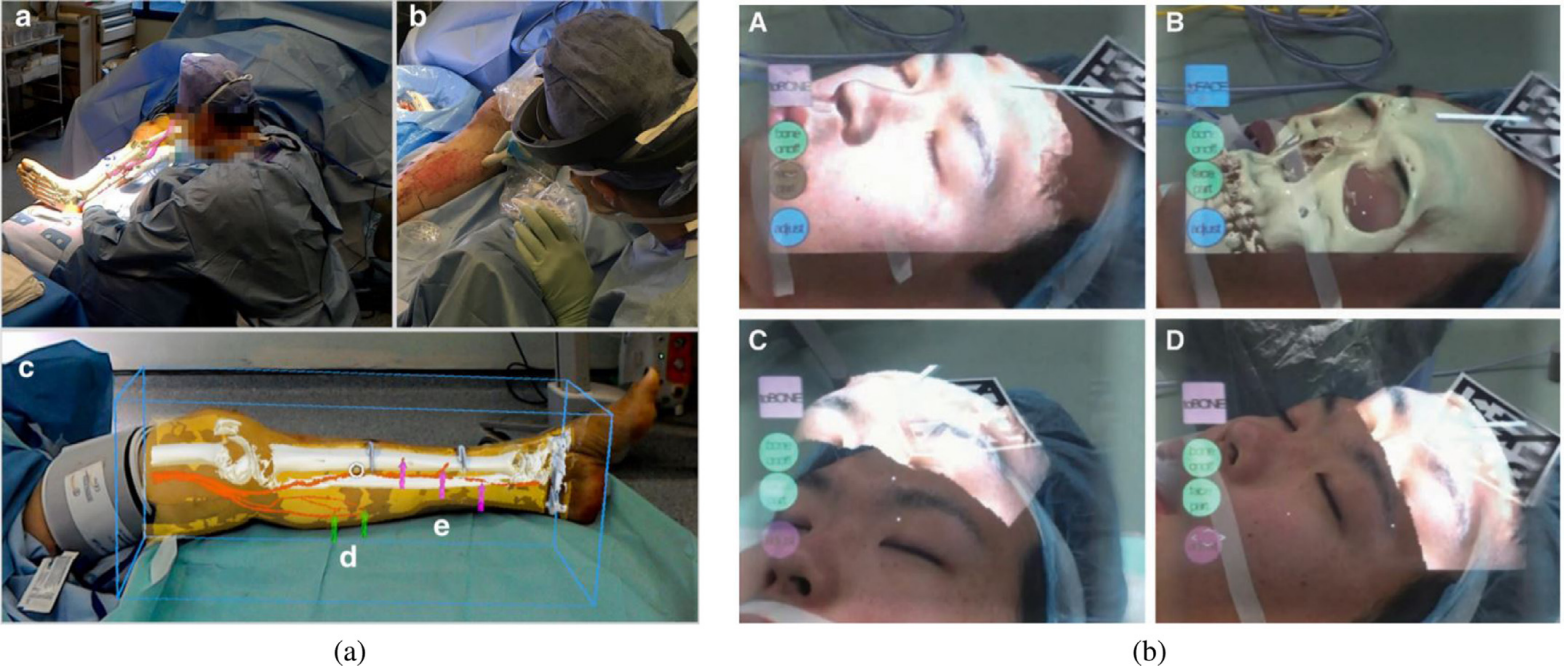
(a) Dissection Guidance example in reconstructive surgery: HoloLens based identification of vascular pedunculated flaps: a CTA-based 3D model of a female patient’s leg consisting of segmented skin, bone, bone, vessels and vascular perforators lower leg is superimposed on the patient anatomy. The surgeon confirms perforator location with audible Doppler ultrasonography *(source:*Fig. 3*ofPratt et al. (2018))* (b) Surgical Anatomy Assessment example in plastic surgery: AR views of the Moverio BT-200 smart glasses showing a patient with osteoma and holographic facial anatomy (face surface and facial bones including the osteoma) superimposed onto a patient’s face *(source:*Fig. 8*ofMitsuno et al. (2017))*
